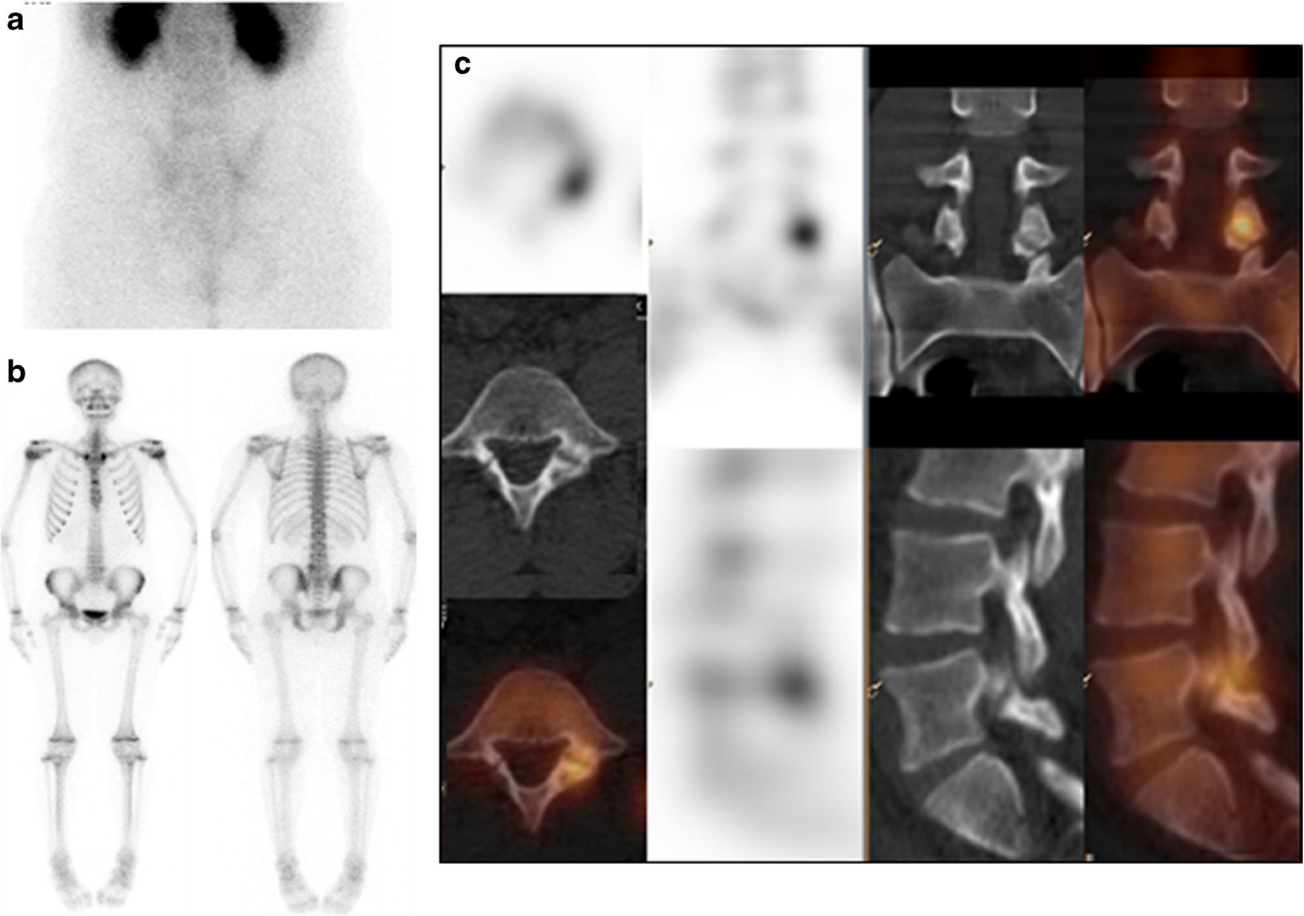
Bone SPECT/CT diagnosis of spondylolysis in an adolescent patient. A14-year-old girl was referred for evaluation of persistent low back pain. Early planar scan of lumbar spine and pelvis (a) shows no hyperaemia. Delayed WB-BS, anterior and posterior views (b) show a focus of abnormal ^99m^Tc-MDP uptake in left aspect of L5 vertebra, localized by SPECT/CT (c) to the left L-5 articular facet, consistent with spondylolysis
